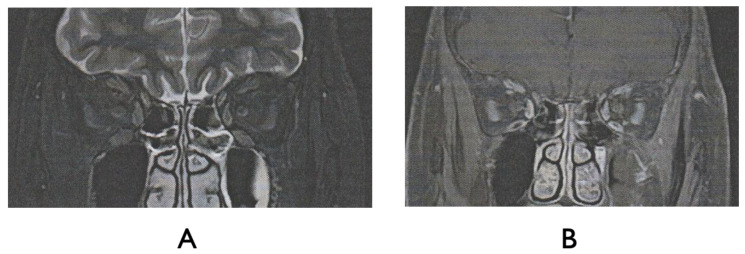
T2 short TI inversion recovery (STIR) (A) and Gadolinium-enhanced MRI (B) findings. No abnormal findings of the optic nerves were found on MRI; as such, there was low suspicion for optic neuritis as the cause of patient’s vision loss and right optic disc swelling.
